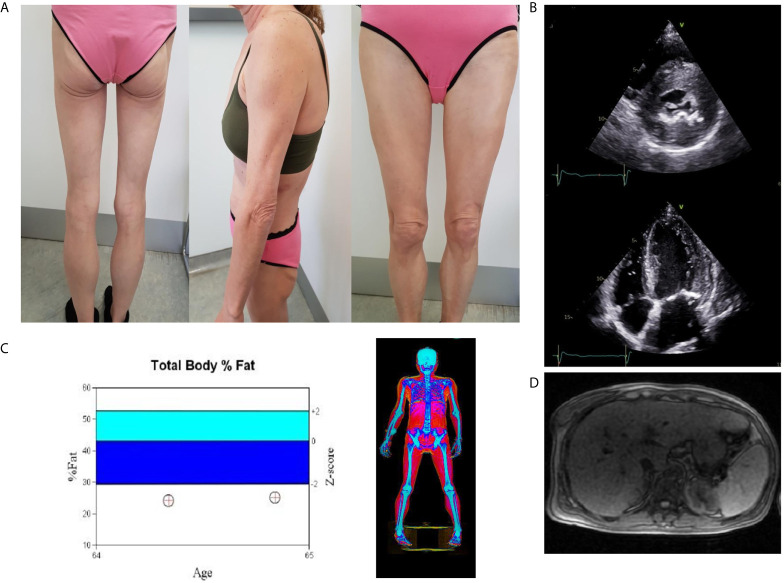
(A) Total lipoatrophy of extremities and gluteal area, (B) Two dimensional echocardiography revealed left ventricular hypertrophy (interventricular septum thickness 20 mm), (C) DXA showed increased fat tissue of the abdominal region and decreased fat mass of the upper and lower limbs. Follow-up DXA indicated unchanged total fat mass content, (D) The abdominal MRI image shows hepatic steatosis.
